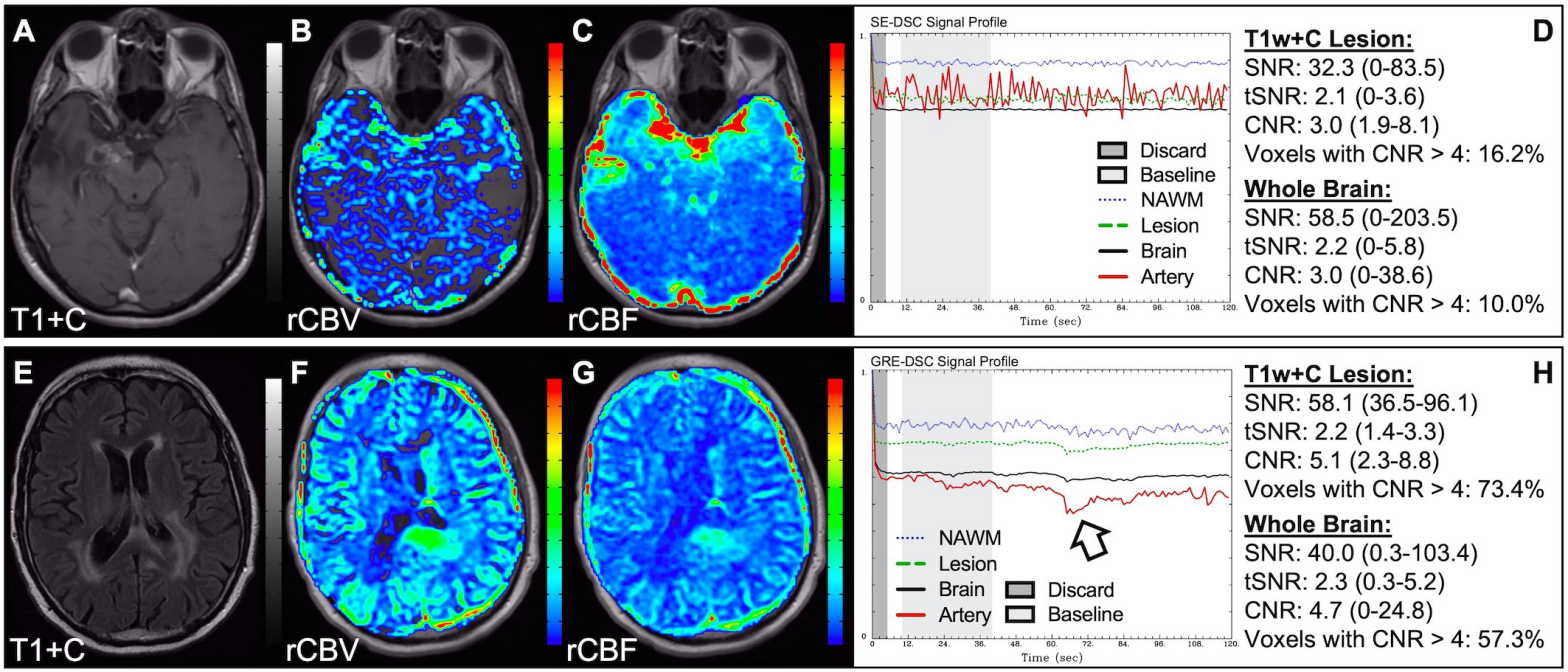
Absent CA changes despite injection. Images are from a 36 y/o male with (pre-WHO 2007) grade 2 astrocytoma (A–D) and a 68 y/o male with WHO 2007 grade 2 astrocytoma (E–H). Pictured are post-CA T1w (A), FLAIR (E), rCBV (B,F), and rCBF (C,G) images and corresponding SE (B–D, TE = 80 ms) and GRE (F–H, TE = 30 ms) DSC signal profiles, where CA was not properly administered due to an IV leak (D) or because the IV line was clamped but allowed some CA to enter (H) This can be recognized by the lack of DSC signal drop in brain and correspondingly low CNR across the brain. While the perfusion data in (F–H) is not trustworthy, follow-up DSC imaging corroborated the finding of hyperperfusion in the T1w+C lesion owing to the robustness of rCBV calculation despite a DSC signal profile resulting from a weak post-bolus CA injection (arrow).
